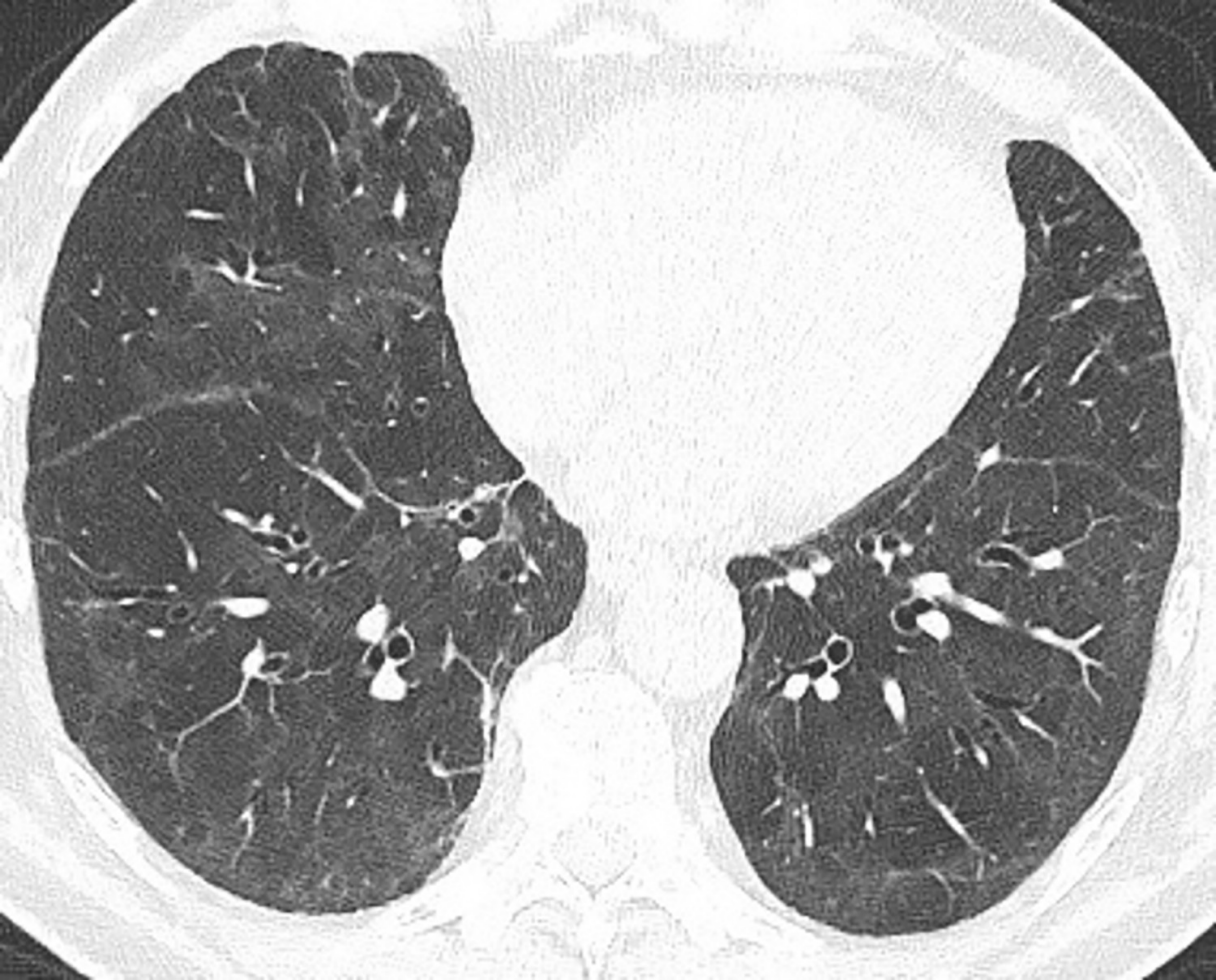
Computed tomography scan 3 months following SARS-CoV2 infection. Bilateral ground-glass opacities and mild peripheral reticular pattern.
